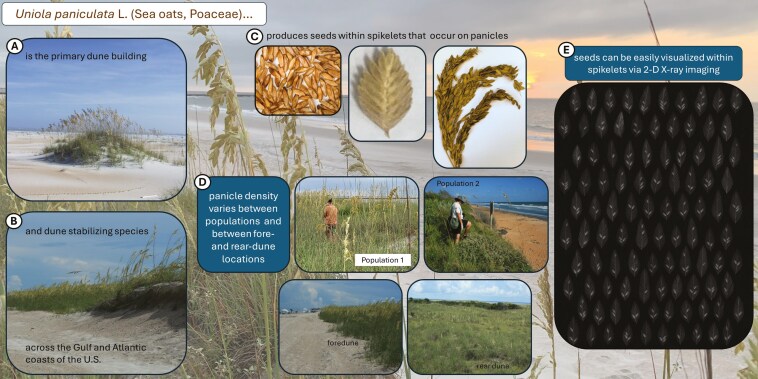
Visual representation of *Uniola paniculata* ecological significance (A, B); From left to right: seeds, spikelets, and panicles (C); variation of panicle density (D); and spikelet X-ray image example (E).
